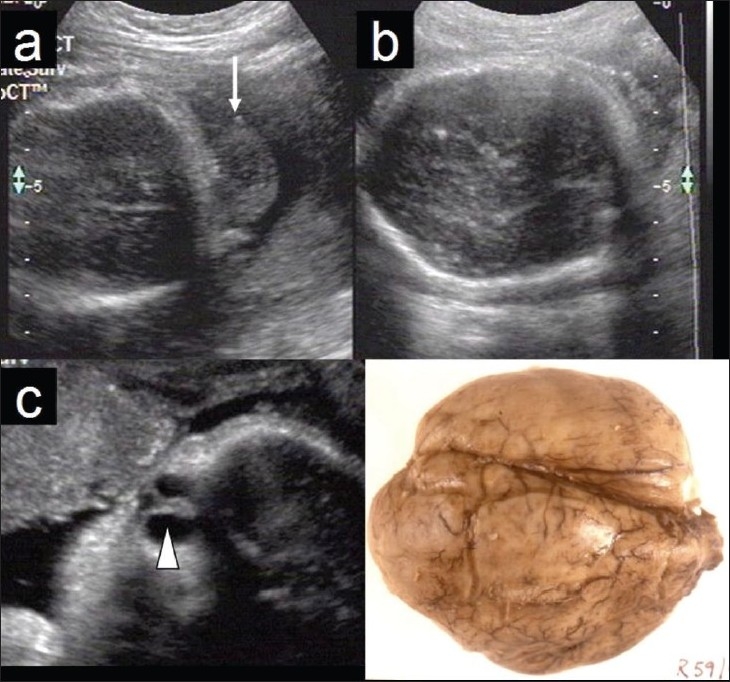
Walker-Warburg syndrome. Axial sections of the fetal cranium show occipital encephalocele (arrow in a) and absent sulci (b). Parasagittal section of the fetal orbit (c) shows retinal detachment (arrowhead). Autopsy picture of the fetal brain (d) demonstrates agyriaWolf-Hirschhorn syndrome: (chromosomal - 4p deletion) Lead clue is severe early-onset IUGR with normal Doppler findings. Other features include ‘Greek helmet’ facial appearance with prominent glabella, rectangular nose, downturned mouth, hypospadias, cardiac and urinary tract defects [Figure 3].
